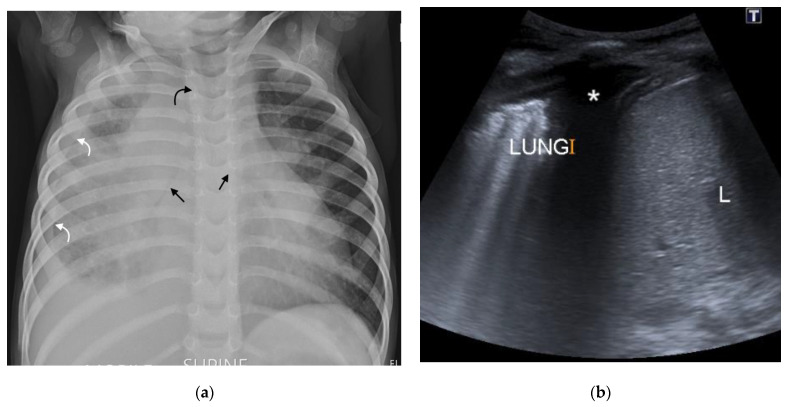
(a,b): (a) Supine AP chest radiograph in a 17-month-old male with PTB demonstrating right sided pleural effusion with veiling of the right hemi-thorax and a lamellar component tracking up along the lateral chest wall (curved white arrows). There is also attenuation of the bronchi bilaterally (straight black arrows), bowing of the trachea from lymphadenopathy (curved black arrow) and an enlarged, globular-shaped heart, consistent with a pericardial effusion (confirmed on ultrasound, not shown here). (b) Chest ultrasound confirming the pleural effusion (star) seen as hypoechoic fluid between the parietal pleura, diaphragm, and right lung.
